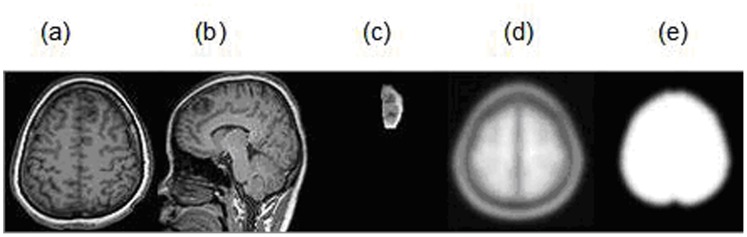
MRI images,mask and template images. (a),(b) axial and sagital structure images of a LGG patient in the left frontal lobe (T1 Flair), (c) masking image of left frontal LGG, (d) T1 template, (e) brainmask in SPM.
